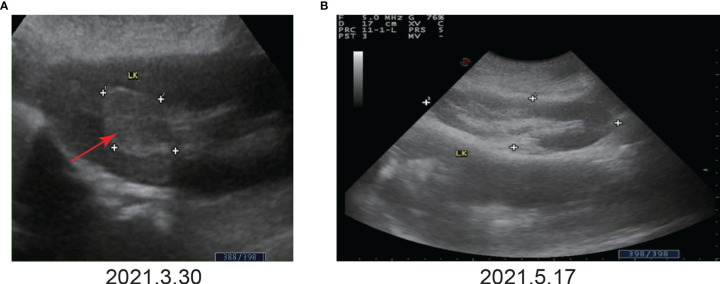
Color Doppler ultrasound before and after immunotherapy. (A) Before immunotherapy. Color Doppler ultrasound showed a space-occupying lesion in the upper calyx of the left kidney, approximately 33 mm×24 mm. (B) After the third immunotherapy. Color Doppler ultrasound showed that no tumor was found.
